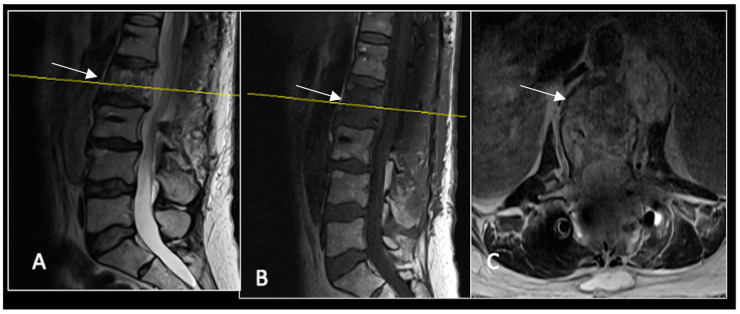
A 6-month postoperative surveillance MRI shows no obvious evidence of residual tumour (arrow) disease progression, but there is significant artefact. The yellow line on the sagittal images denotes the level of the axial image. (A) T2W sagittal; (B) T1W sagittal; (C) T2W axial.
